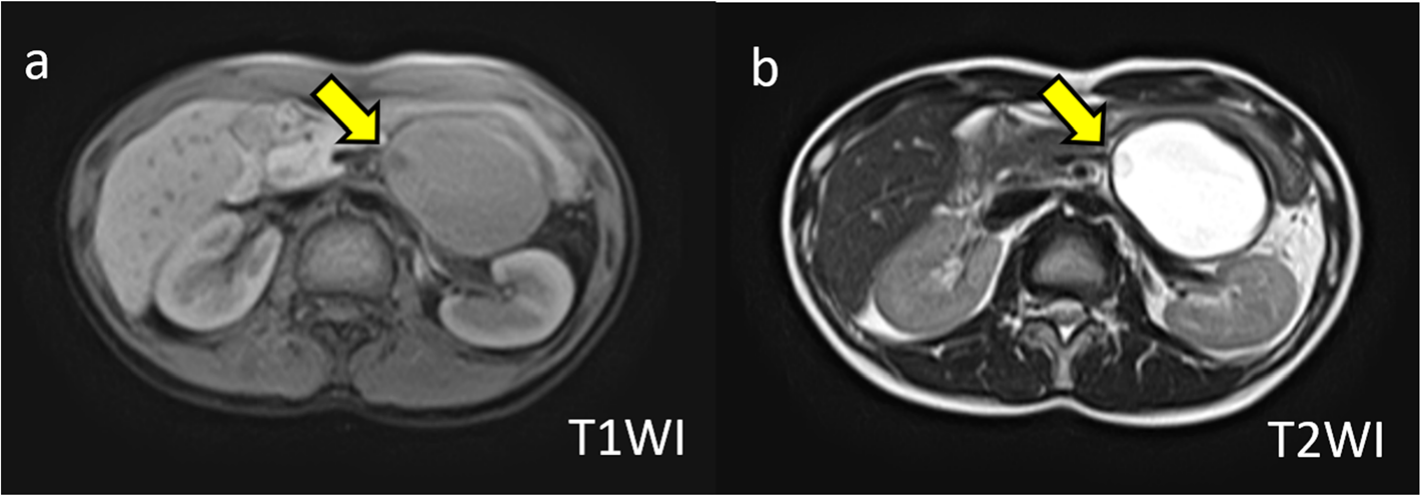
a T1-weighted image. A cystic tumor with an internal septal formation was present in the pancreatic tail. The cystic lesion showed a hypo-intense signal with nodular lesion (arrow). b T2-weighted image. The cystic lesion showed a hyperintense signal with nodular lesion (arrow)
